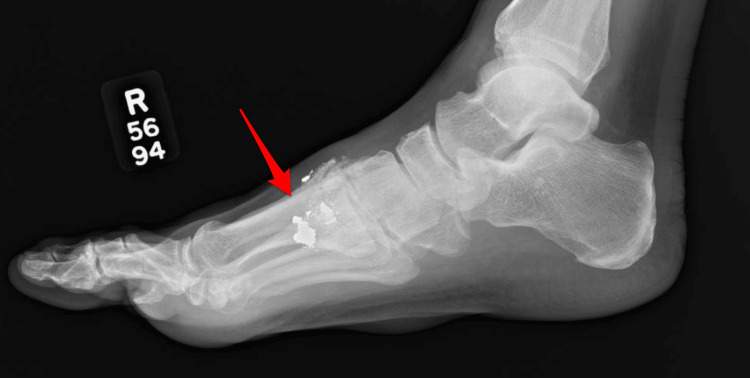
Right foot X-ray with embedded BB pellet fragments.
